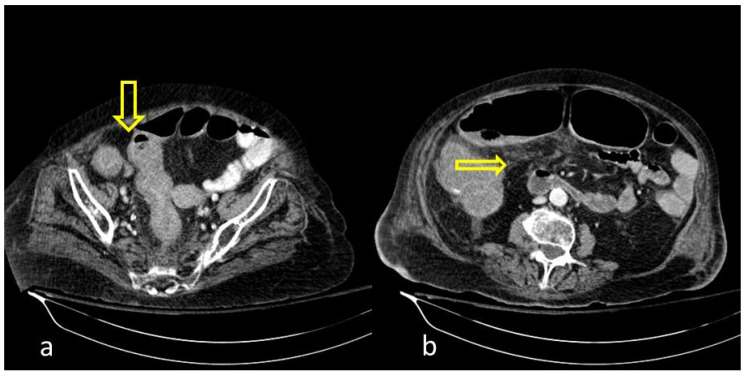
COVID-19 (+) hospitalized 75-year-old male patient with abdominal pain and diarrhea. Abdominal Contrast Enhanced CT (CECT) depicted bowel wall thickening ((a), thick arrow) and mesenteric fat stranding ((b), thin arrow). The imaging findings are indicative of inflammatory colitis.
